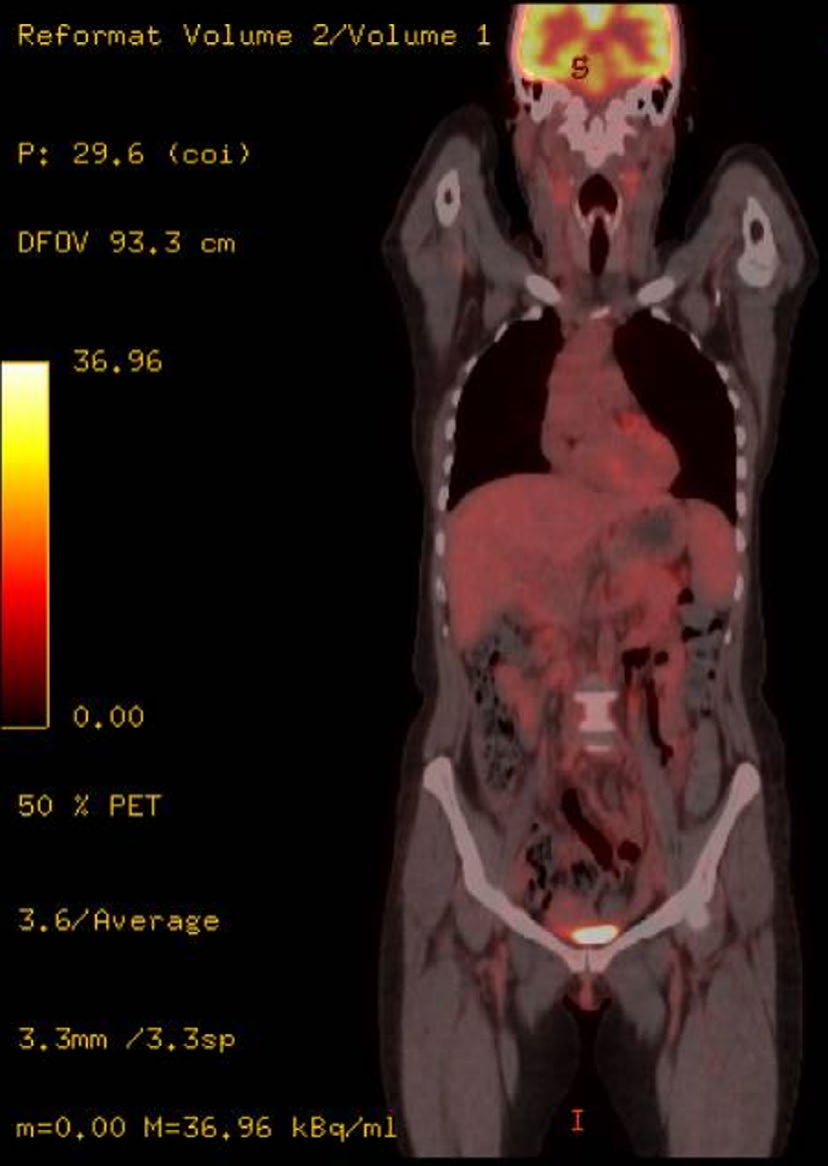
CT/PET scan after BV treatment
